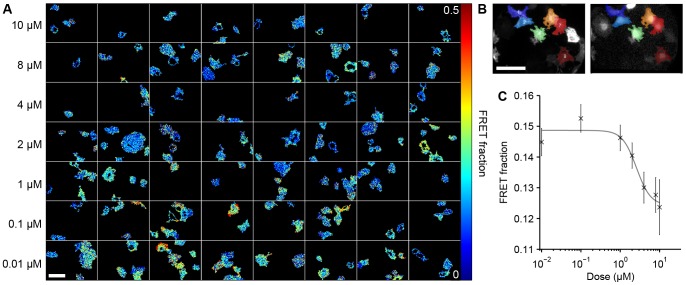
Global analysis of an IPA-3 dose-response dataset modulating the interaction between Rac1 and Pak1. Global analysis was applied to a multiwell plate dose-response dataset showing the effect of the inhibitor IPA-3 on interaction between Rac1 and Pak1 using an mTurquoise variant of the FLAIR biosensor in COS-7 cells stimulated with EGF. A) representative images from each inhibitor concentration showing distribution of fraction undergoing FRET. B) examples of automatic image segmentation with *(left)* donor intensity and *(right)* acceptor images shown in grey-scale with coloured segmented cell regions overlaid. *C*) plot of fraction of donor molecules undergoing FRET against IPA-3 concentration, averaged across segmented cells with fitted dose-response curve. Error bars indicate 95% confidence intervals on average FRET fraction over segmented cells at each dose. White scale bar represents 100 µm.
